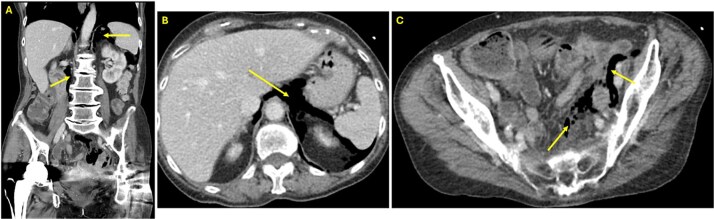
(A) Coronal view CT of the abdomen and pelvis showing free air in the retroperitoneum (arrows). (B) Axial view CT of the abdomen and pelvis at the level of the liver demonstrating free air in the retroperitoneum (arrow). (C) Axial view CT in the pelvis revealing free air in the colonic mesentery (arrows), consistent with extraluminal gas from rectal perforation.
